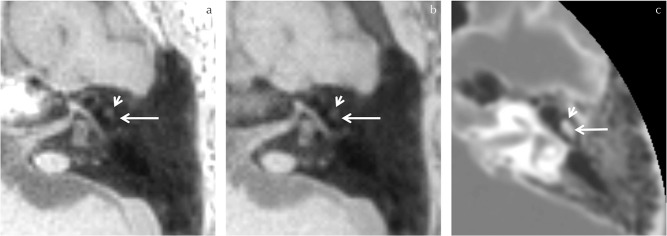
A 64-year-old woman. PETRA image without fat suppression pulses (a), PETRA image with fat suppression pulses (b) and the corresponding CT image (c). The head of the malleus (short arrow in each image) and the body of the incus (long arrow in each image) are similarly visualized by PETRA with and without fat suppression pulses. The signal of the ossicles appears to come not from fat, but from water. CT, computed tomography; PETRA, pointwise encoding time reduction with radial acquisition.
